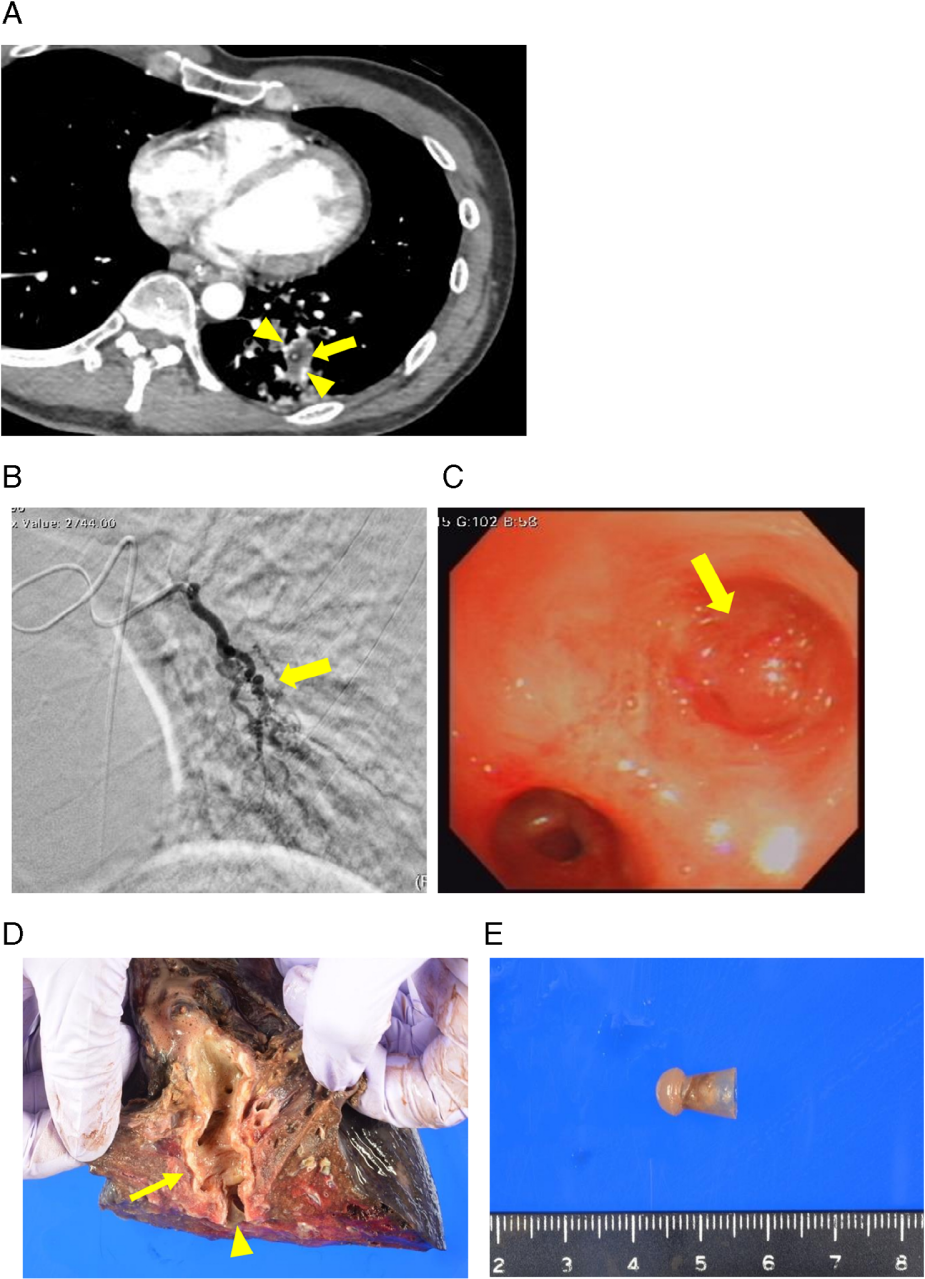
(A) Early phase of contrast‐enhanced computed tomography showing a foreign body (FB) in the lateral basal bronchus (arrow) surrounded by enhanced bronchial artery dilatation (arrowhead). (B) Bronchial angiography showing a meandering and markedly dilated (arrow) bronchial artery feeding the left lower lobe (LLL). (C) Bronchoscopy performed at the level of the left lower bronchus showing excessive granulation tissue formation and persistent oozing around the FB in the lateral basal bronchus (arrow). (D) Cross‐section of the left inferior lobar bronchi (arrow). The FB was retrieved from the lateral basal bronchus (arrowhead). (E) The shape of the FB was very unique, and its surface was translucent, with a texture similar to that of plastic or rubber.
